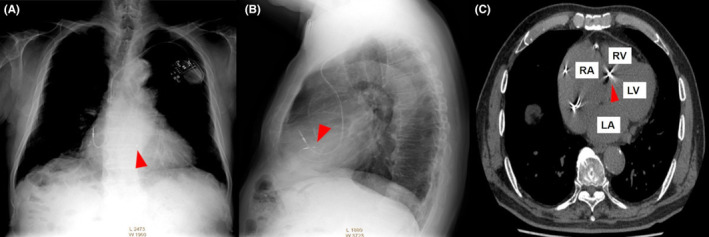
Chest X‐ray (A, B) and CT (C) showing the RV lead implanted into the basal RV septum (arrowheads). CT, computed tomography; RV, right ventricular.
